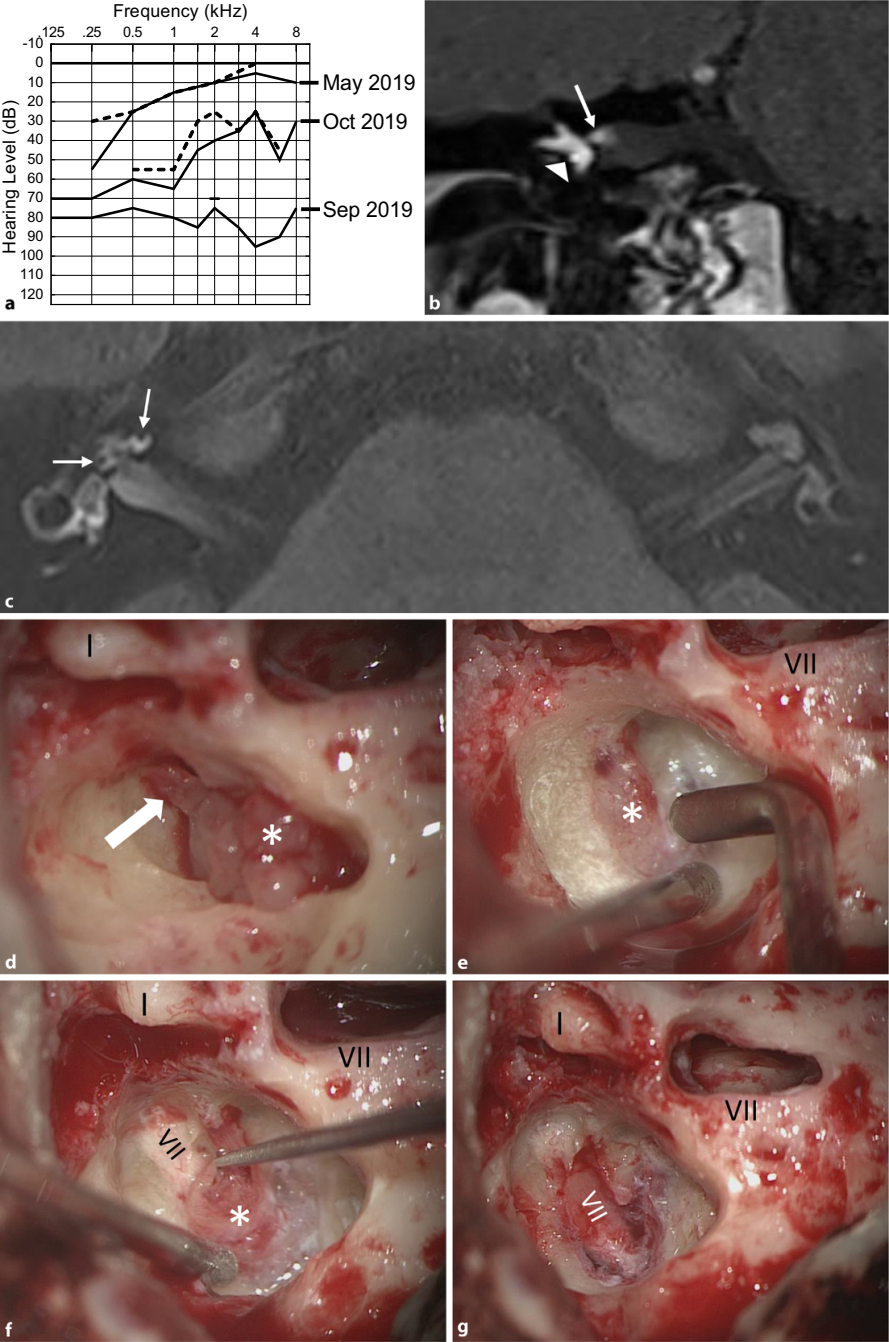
Transmacular ISL (patient 8 in Table 2). a Pure-tone audiogram showing fluctuating hearing loss (*full line*: air conduction, *dashed line*: bone conduction) most likely based on a secondary cochlear hydrops because of the tumor in the vestibule (c). b MRI (coronal, T1-w + Gd) showing the tumor in the right vestibule (*triangle*), the ampullary ends of the superior and lateral semicircular canals and extension along the superior vestibular nerve into the IAC (→). c MRI with hydrops sequence (3D inversion recovery, 6 h after systemic Gd application) showing a mild cochlear hydrops (→). d Intraoperative view with the tumor (*asterisk*) in the vestibule and along the superior vestibular nerve towards the fundus of the IAC (→). e–g The tumor (*asterisk*) is gradually separated from the facial nerve under facial nerve monitoring control. *VII* facial nerve, *Gd* gadolinium, *I* incus body, *w* weighted
